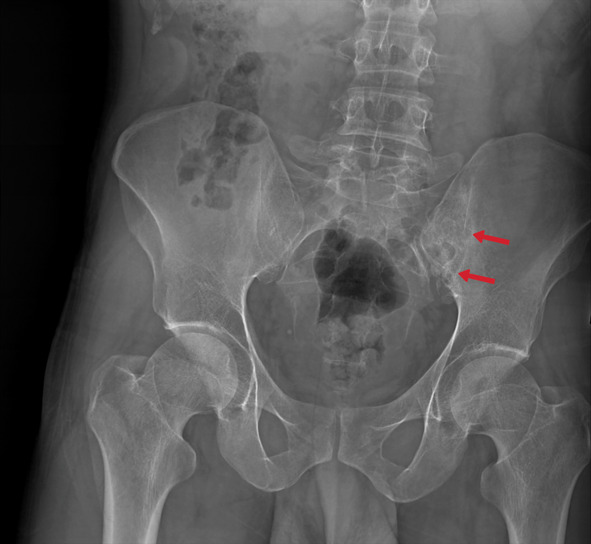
Pelvic x-ray of a 46-year-old male. The late finding of tuberculous sacroiliitis is characterized by degenerative changes in the left sacroiliac joint (arrows)
